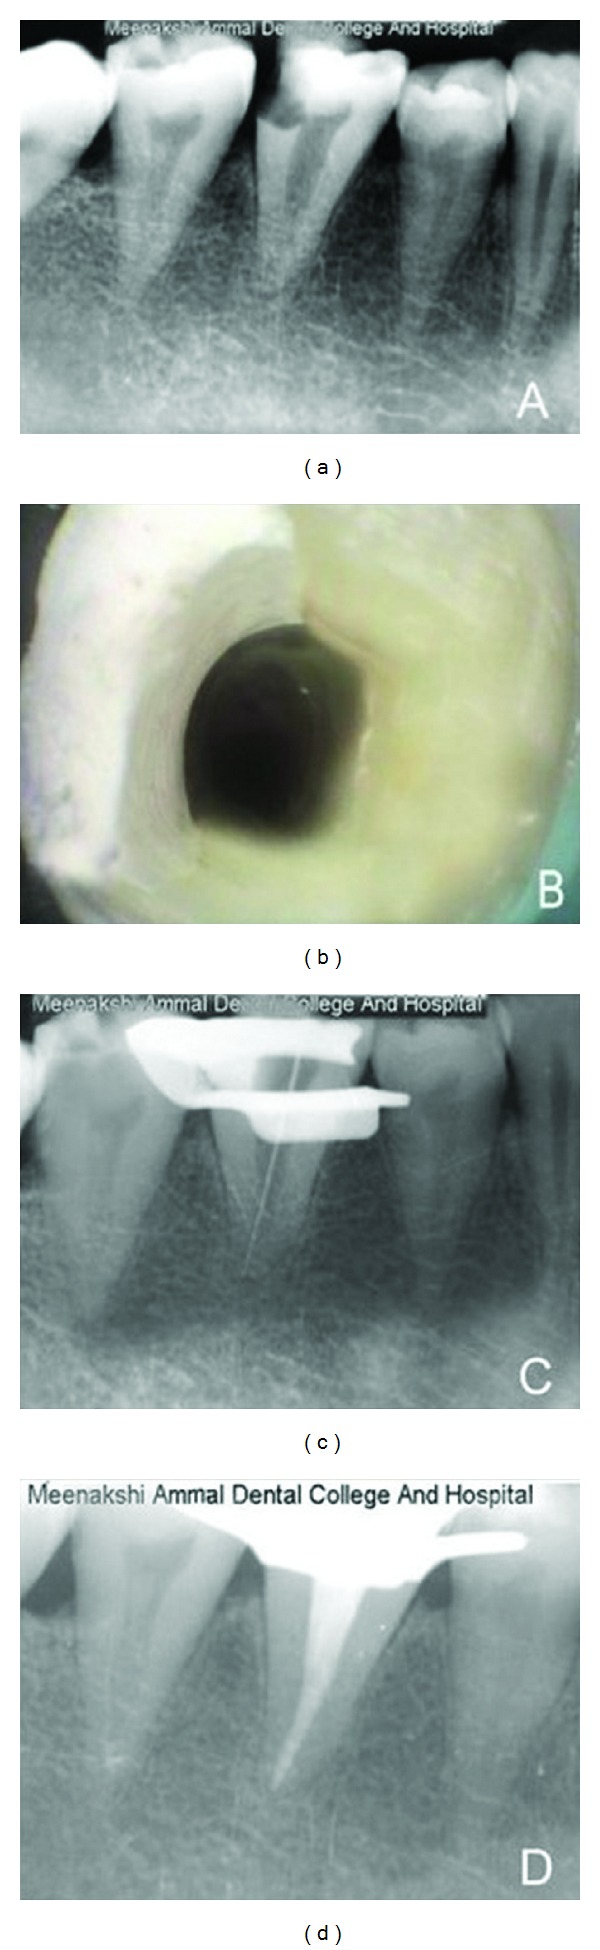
(a) Preoperative radiograph showing the posterior teeth with single root. (b) Access opening demonstrating single canal. (c) Working length radiograph. (d) Postobturation radiograph.
